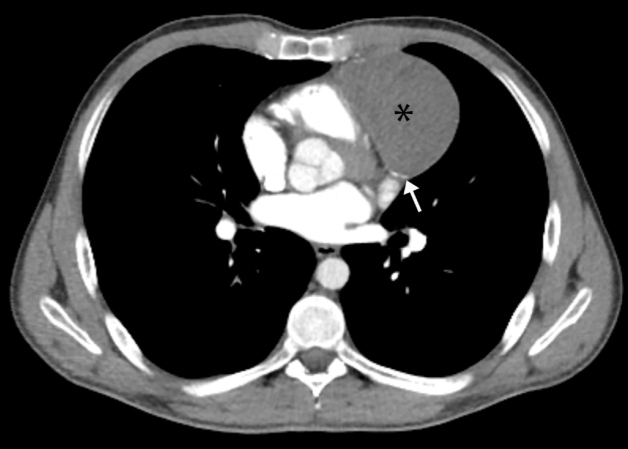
Axial contrast-enhanced computed tomography. There is a pericardial heterogeneous cystic mass (asterisk) containing peripherally located foci of calcifications (arrow).
